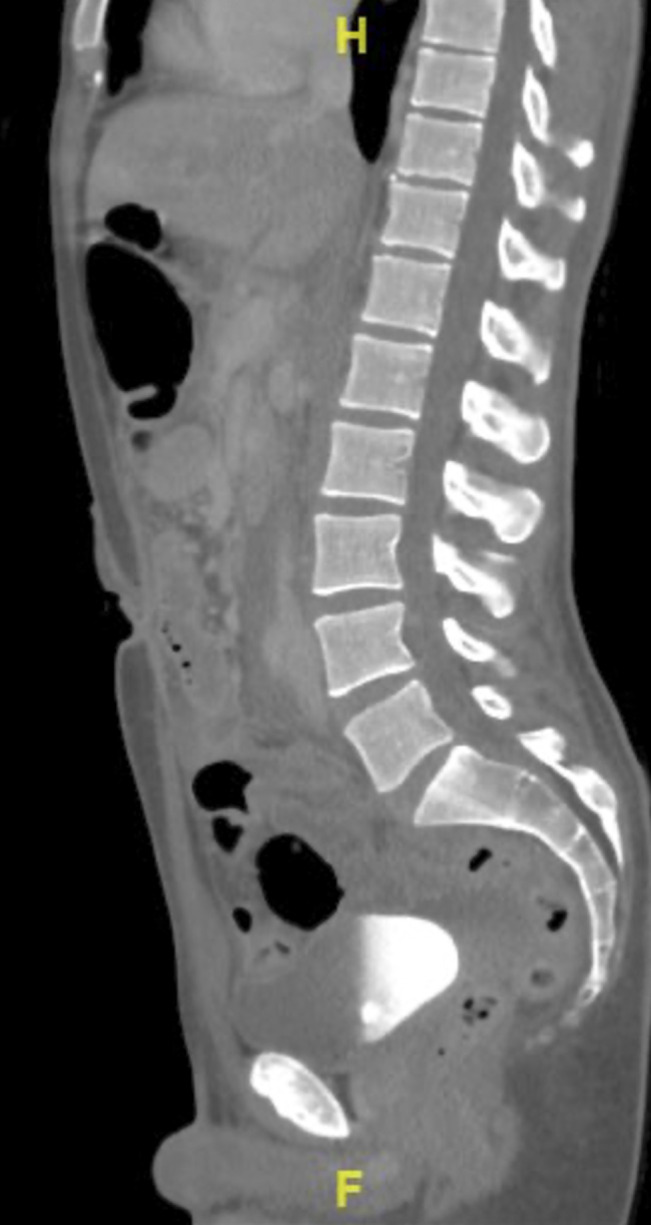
Sagittal and axial CT cuts showing a 2.4 × 8.6 cm retroperitoneal hematoma but no abnormality in the spine at L3-4.
